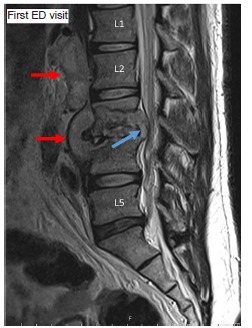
Pre-operative sagittal magnetic resonance imaging from index visit demonstrates bony destruction of third and fourth lumbar vertebrae. A large epidural abscess extending from first to fourth lumbar vertebrae (red arrows) projects through third lumbar vertebral body and compresses the spinal nerve roots (blue arrow). Lumbar vertebrae 1 (L1), 2 (L2), and 5 (L5). *ED*, emergency department.
